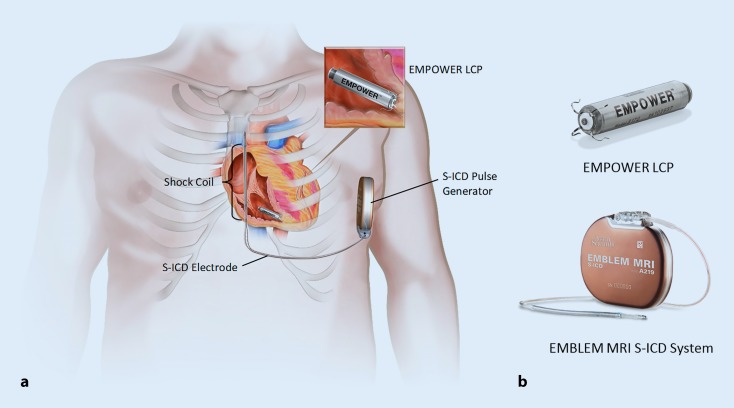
The modular cardiac rhythm management (mCRM) system. a Depiction of implanted mCRM system. b Close-up images of EMPOWER and EMBLEM MRI devices (not to scale). *S-ICD* subcutaneous implantable cardioverter–defibrillator, *LCP* leadless cardiac pacemaker. © 2017 Boston Scientific Corporation or its affiliates. All rights reserved. Used with permission of Boston Scientific Corporation
